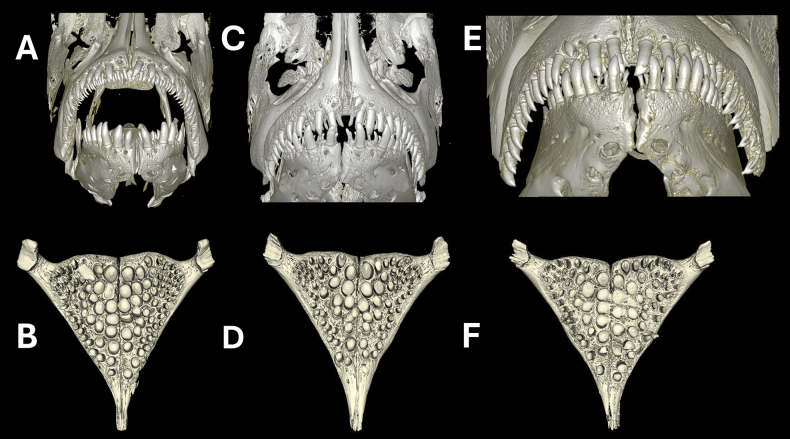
CT scans of the frontal aspect of oral jaws (top) and occlusial aspect of the lower pharyngeal jaws (bottom) of *Labrochromismawe* sp. nov. and *L.mawepili* sp. nov. All are males. From top left, A, B (NMBE 1111873) *L.mawe* sp. nov., Python Island C, D (NMBE 1111893) *L.mawe* sp. nov., Kissenda Island E, F (NMBE 1111906) *L.mawepili* sp. nov., Makobe Island.
